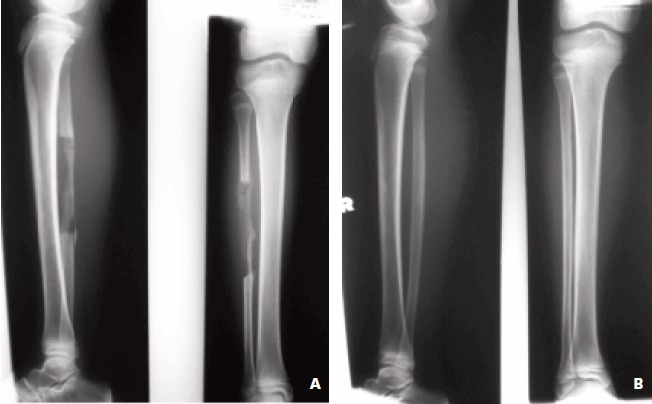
X-ray of the donar fibula site. Picture A shows sign regeneration of fibula at the donar site after few months. Picture B shows
